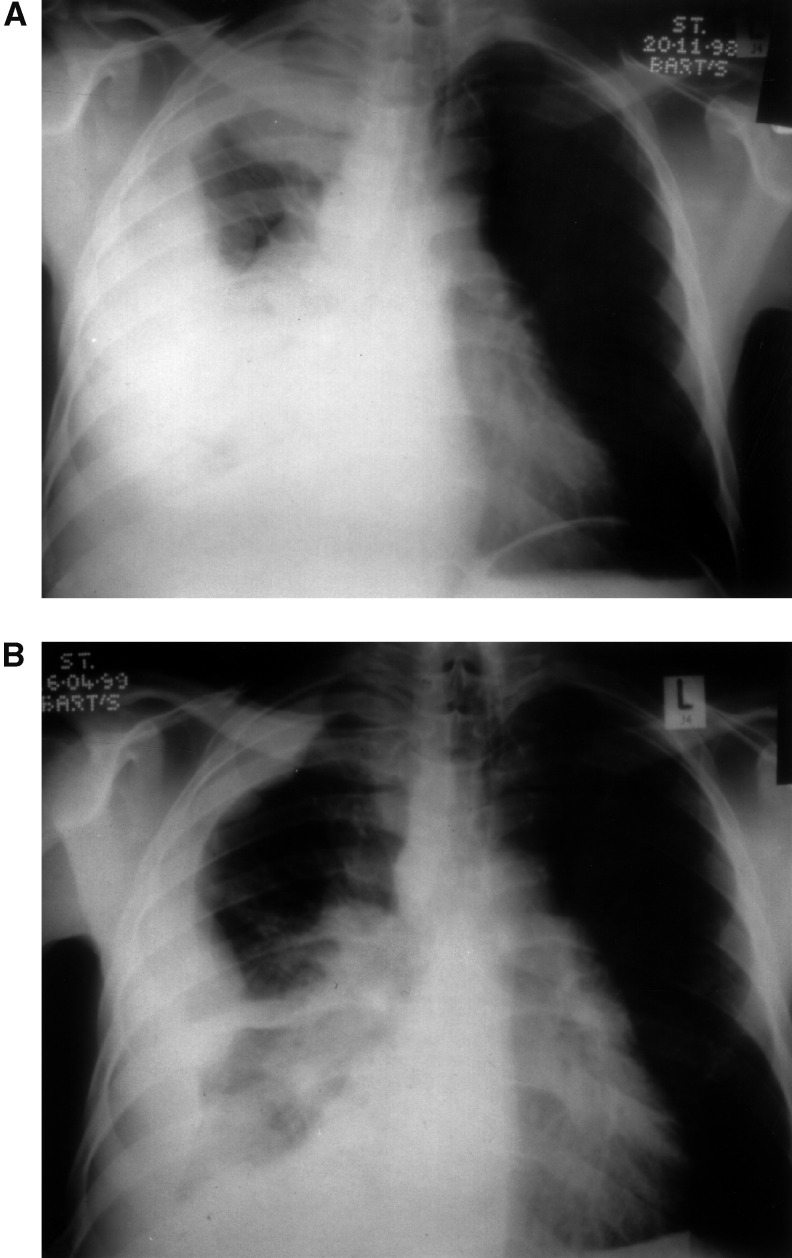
Chest radiograph prior and post-IPM therapy.
